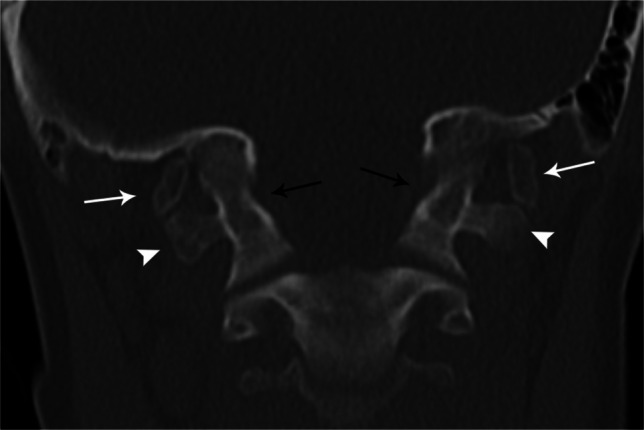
Coronal bone window CT of the spine in a 15-year-old male with bilateral unfused paracondylar processes (*arrows*) with pseudoarticulation with the transverse processes of C1 (*arrowheads*). Note concomitant atlanto-occipital assimilation (*black arrows*)
